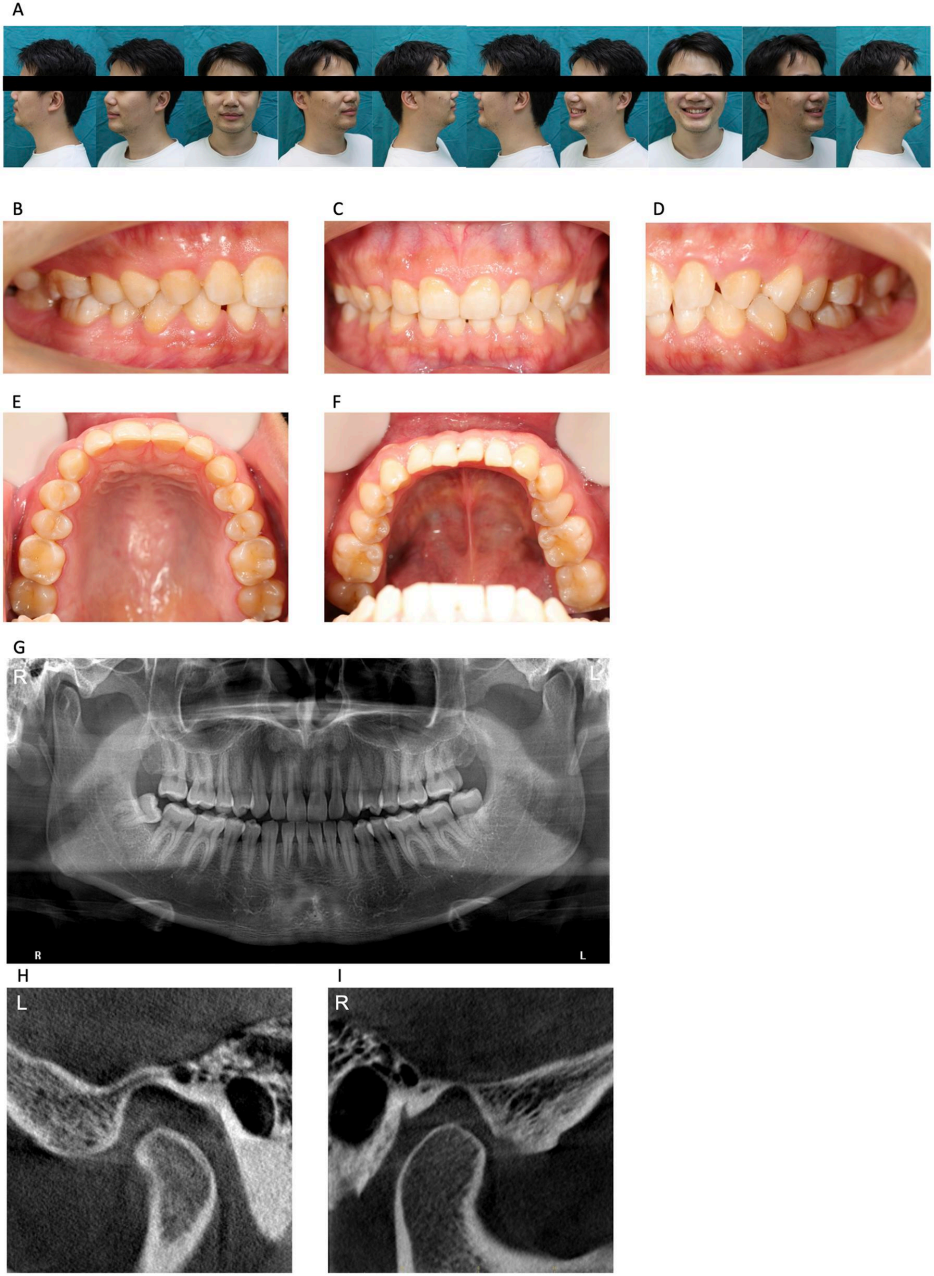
Clinical and imaging examinations of the patient. (A) Photos of the patient at rest and smiling in both anteroposterior and lateral positions; (B) The patient's right occlusal condition; (C) The patient is in a median occlusal state; (D) The patient's left occlusal condition; (E) Intraoral photograph of the patient's upper jaw; (F) A photo of the patient's mandibular opening; (G) Curved surface tomography imaging examination of the patient; (H) CBCT examination of the patient's left temporomandibular joint; (I) CBCT examination of the patient's right temporomandibular joint;.
